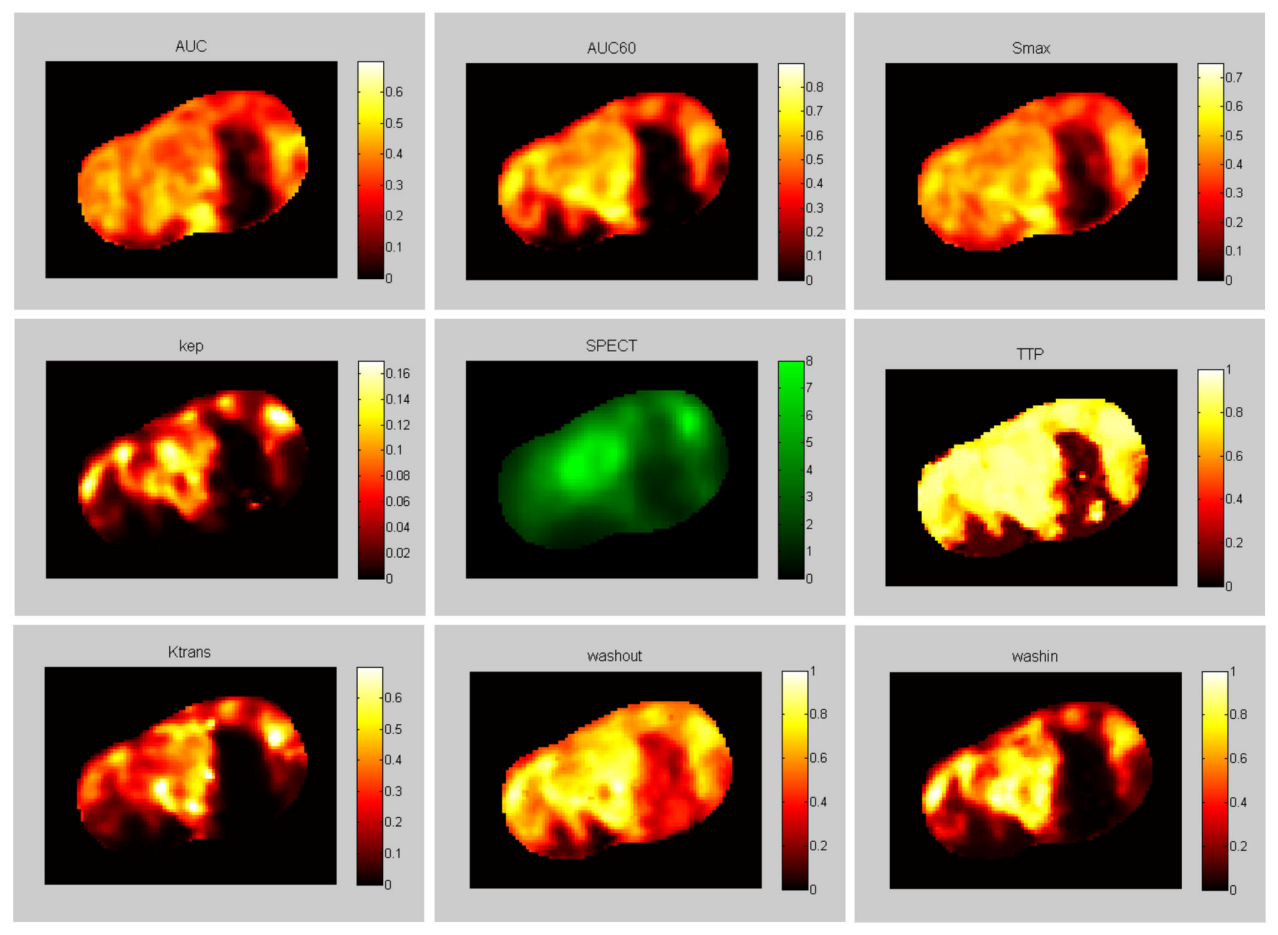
Visualization of the correspondence between SPECT-peptide uptake and DCE-MRI-derived parameters. Color coded images of SPECT peptide uptake (in Bq) (green) and different DCE-MRI parameters (red) for one slice through the tumor. Clockwise starting from the top left: AUC, area under the whole curve; AUC60, area under the curve for the first 60 s; Smax, maximum enhancement; TTP, time to peak; wash-in, slope between the 10% point and the 90% point on the enhancement curve [19]; wash-out [19]; K^trans^ (s^-1^) capillary transfer constant [20]; k_ep_ (s^-1^), contrast exchange rate [20]. All semi-quantitative parameters were normalized and TTP values were inverted for visualization purposes.
